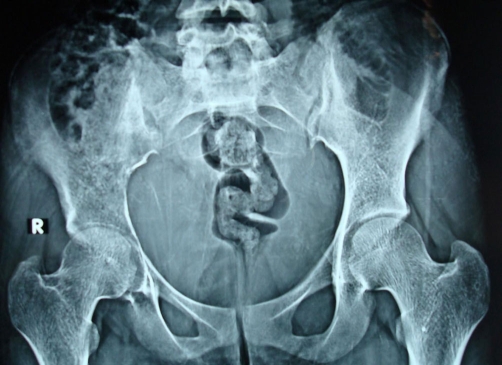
Digital X-ray pelvis AP view showing reduced right hip joint space with displaced fat planes suggestive of effusion.
